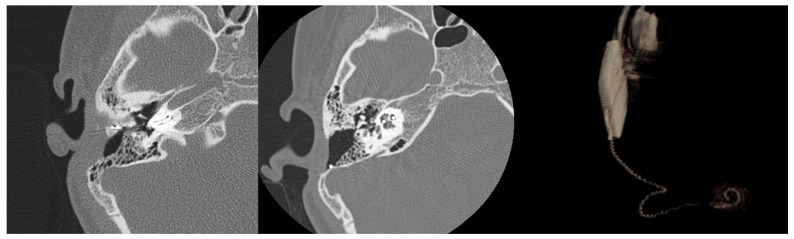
CT scan images depicting the positioning of the old electrode array CI622 (left image) and the new one after reimplantation with a slim modiolar CI632 (middle and right images). The reconstruction (via volume rendering) of the electrode within the cochlea facilitates visualization of the proper positioning of the new electrode in the scala tympani.
